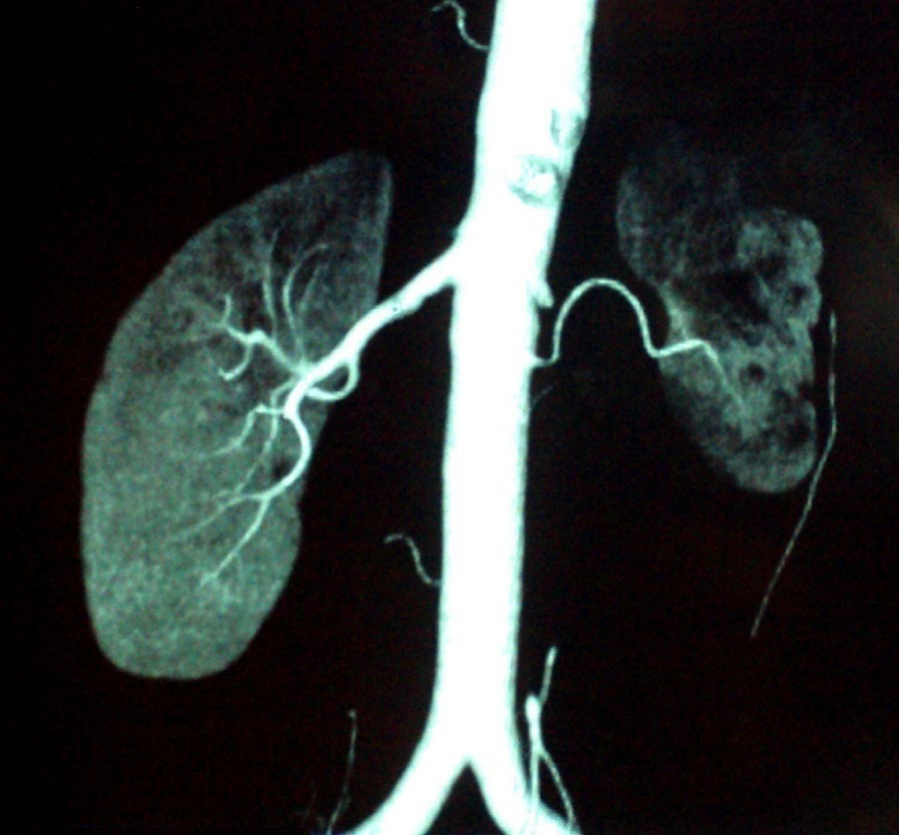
Close-up of CT angiogram showing significant left sided renal artery stenosis, with an atrophied left kidney, which is supplied by an accessory renal artery. There is normal perfusion and function of the right kidney
